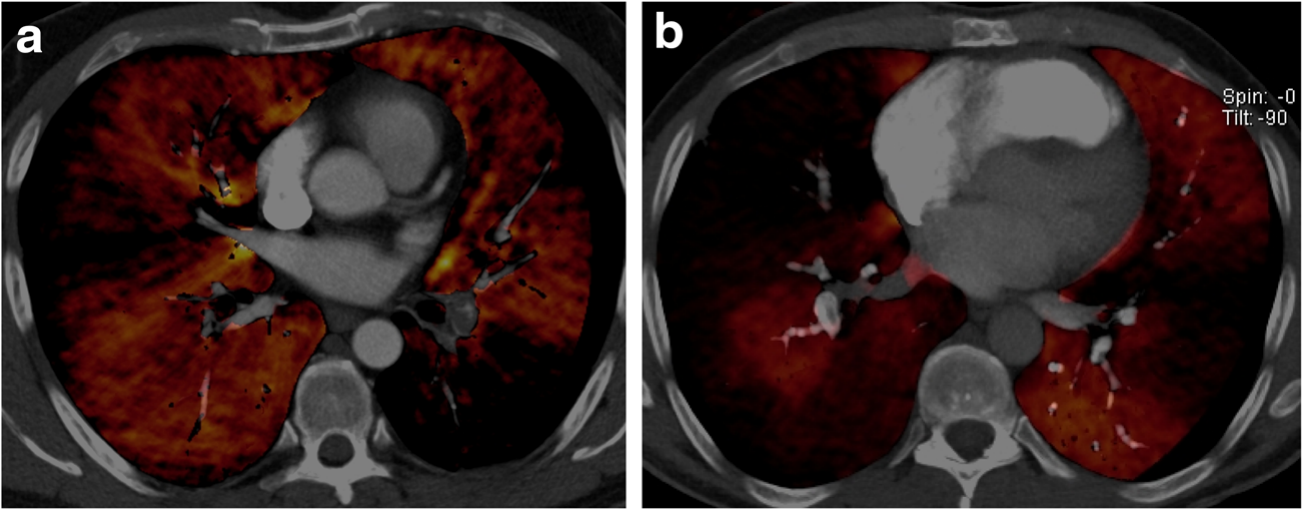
Colour-coded CT iodine map shows a greater extent of perfusion defects (black areas) in CTEPH (b) rather than in CTEPD (a)
